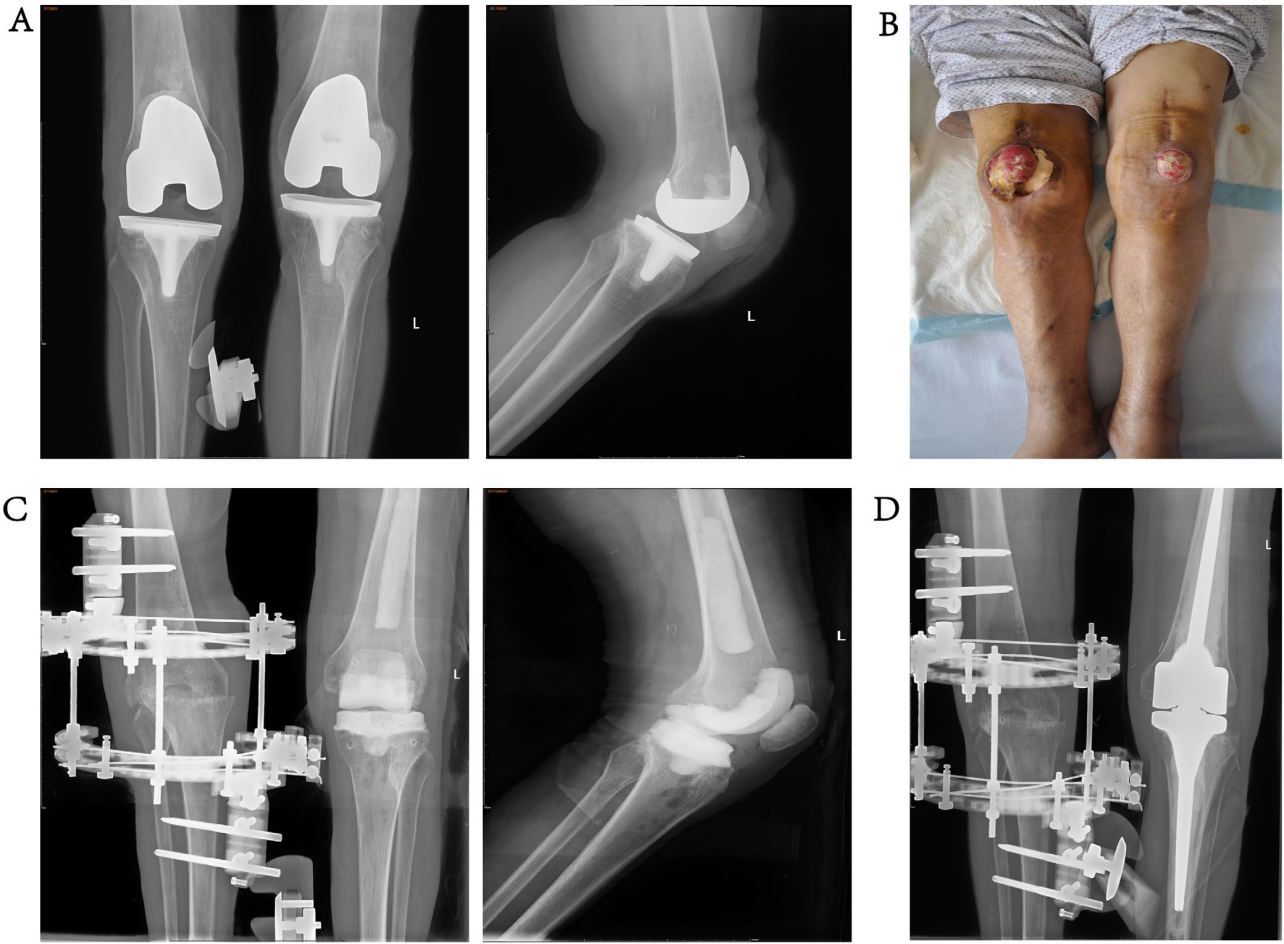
Case images of treatment following infection after TKA: (A) radiograph after primary TKA; (B) clinical appearance of the knee after infection; (C) postoperative radiograph after the first surgery for infection treatment; and (D) radiograph after implantation of a left RHK prosthesis.
